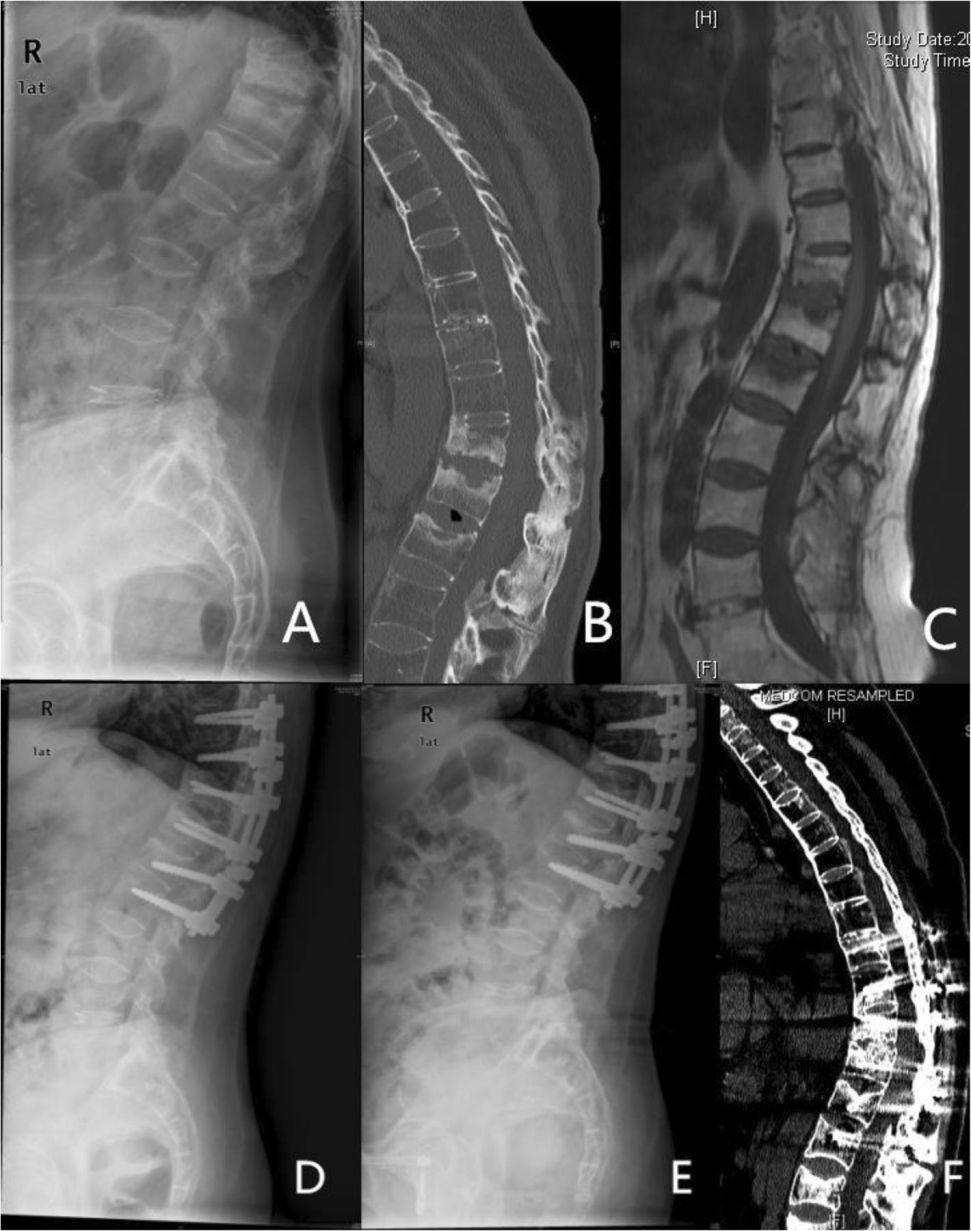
A 61- year old female suffered from progressive kyphosis and mechanical pain for 1 year. Sagittal X-ray of the lumbar spine (a) showed a discovertebral destructive lesions at T10/11. A sagittal reconstructed CT image and MRI (b and c) showed central osteolysis and surrounding sclerotic endplate at T10/11. Sagittal X ray of lumbar spine after the operation (d) showed good internal fixation and kyphosis correction without anterior fusion. Sagittal X ray of lumbar spine (e) and sagittal reconstructed CT image (f) at 2-year follow-up confirmed good fusion of the pseudarthrosis at T10/11
